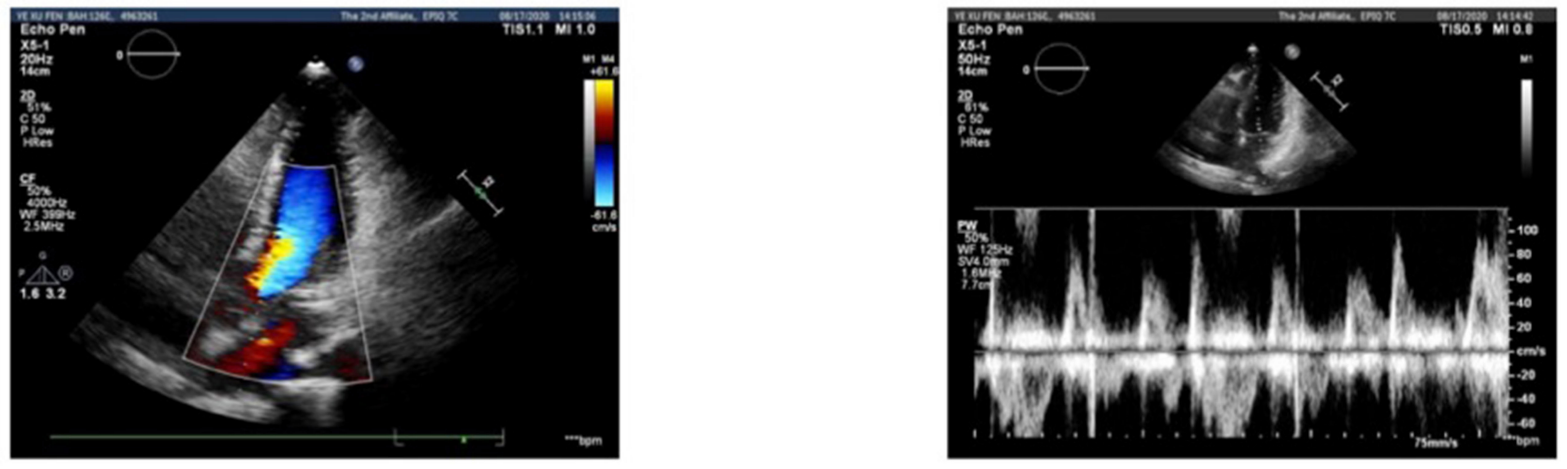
Doppler echocardiogram recordings of blood flow during sinus rhythm and premature ventricular complexes (PVCs).
